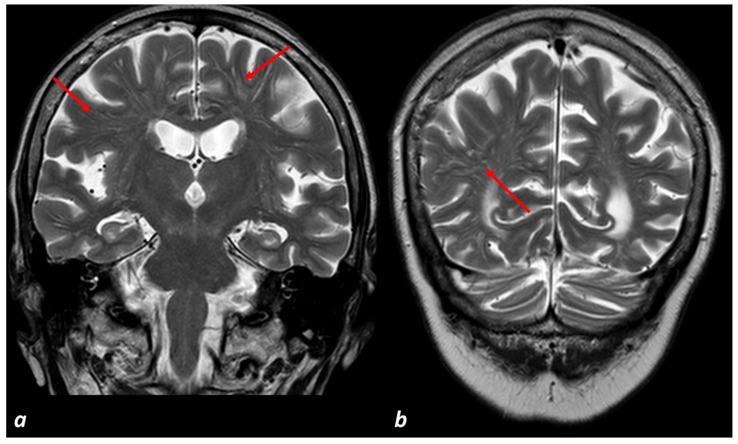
Multiple EPVSs in the CSO and the subcortical WM in T2W-MRI in the coronal plane at the hippocampi (a) and occipital poles (b) level. The red arrows point to the EPVSs.
